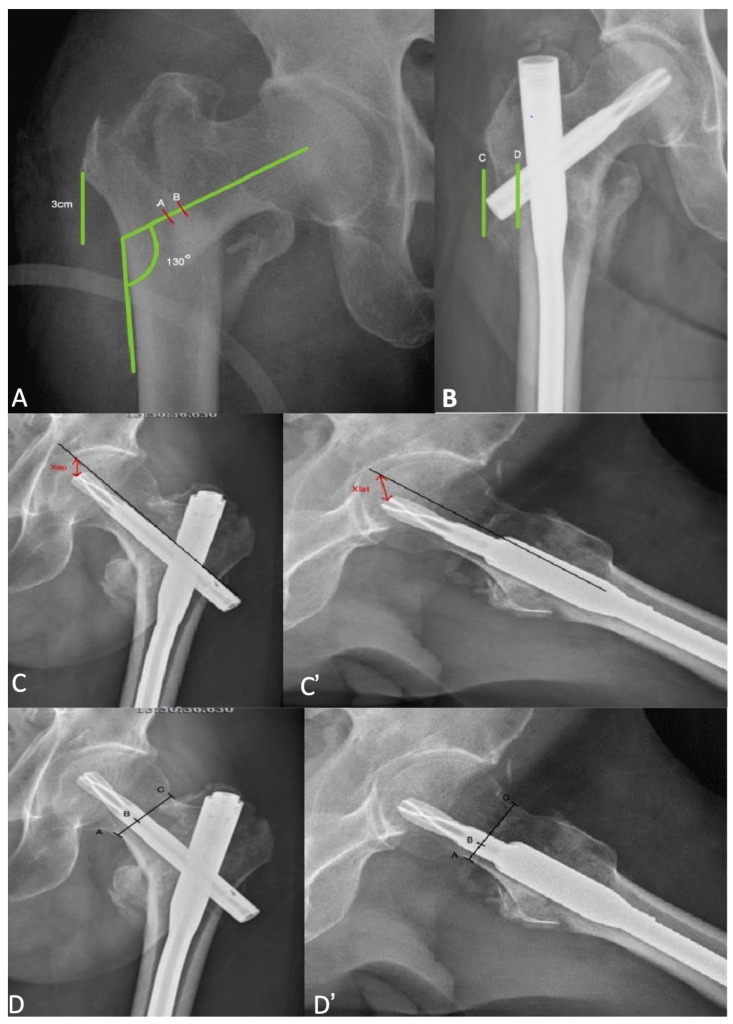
(A) Lateral wall fracture gap length defined as the distance in millimeters from a reference point 3 cm below the innominate tubercle of the greater trochanter, angled at 130° upward to the fracture line (distance between A and B). (B) Distance between the posterior tip of the blade and the lateral cortex (distances C and D). (C,C’) TAD was defined as measurement of tip apex distance and is the sum of the distance from the apex of the femoral head to the top of the lag screw on anteroposterior and lateral radiographs (Xap + Xlat). (D) Parker’s ratio in AP% was AB/AC. (D’) Parker’s ratio in lateral% was AB/AC.
